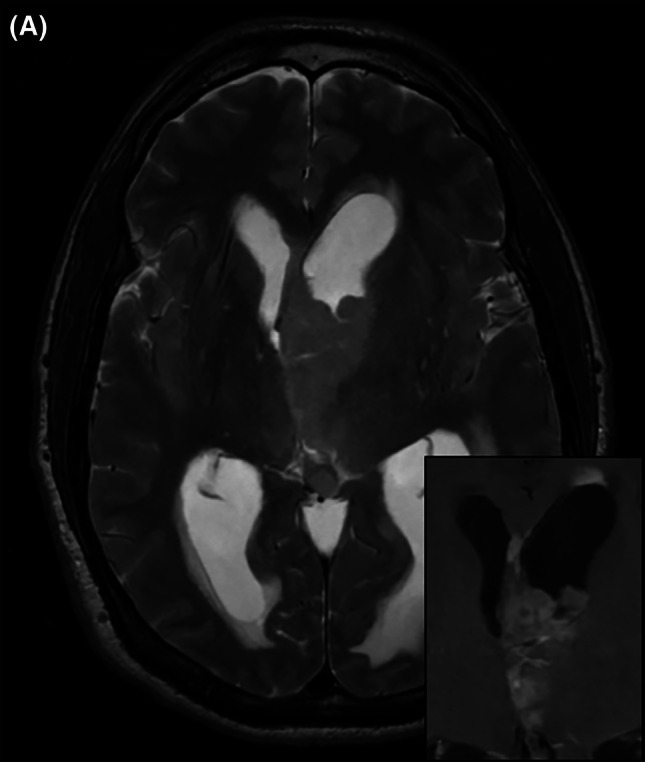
Preoperative MRI: Axial T2 3D fast‐recovery fast spin‐echo imaging demonstrates a large intraventricular mass, hydrocephalus, and transependymal edema (A). The tumor exhibits heterogeneous enhancement on T1 post‐contrast sequence (inset).
